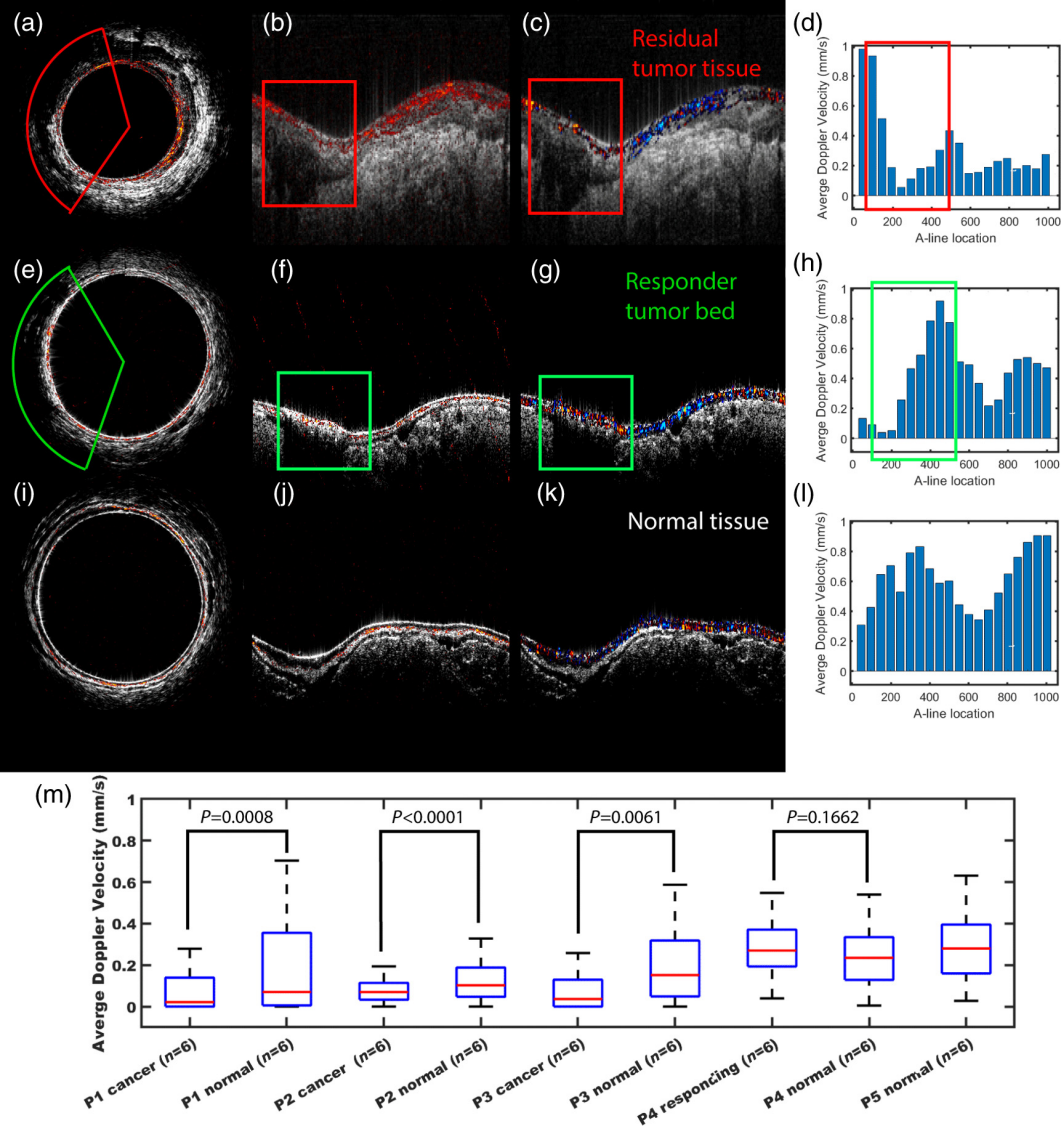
Examples of PA images coregistered with US, Doppler coregistered with US, and flow speed across a rectal cross-section. (a) Polar and (b) linear US coregistered with PA scan of a patient with cancerous tissue bordered in the red rectangle. (c) US coregistered with a Doppler scan of the same patient (d) Doppler flow speed bar chart across rectal tissue of the same patient, with cancerous tissue bordered in red. (e) Polar and (f) linear US coregistered with a PA scan of a patient with treatment scarring, bordered in green (g) US coregistered with Doppler scan of the same patient with treatment scar, labeled in green (h) Doppler flow speed bar chart across rectal tissue of patient with treatment scar, labeled in green (i) polar and (j) linear US coregistered with PA scan of patient with normal rectal tissue (k) US coregistered with Doppler scan of the patient with normal tissue (l) Doppler flow speed bar chart across rectal tissue of the same patient. (m) Box plot of average Doppler velocity across patients, analyzed with a t-test, the cancer regions exhibit statistically significant lower velocity than normal regions in all three cancer patients (P1–P3), and the responding tumor bed region exhibits slightly higher flow velocities than normal regions, but these are not statistically significant.
